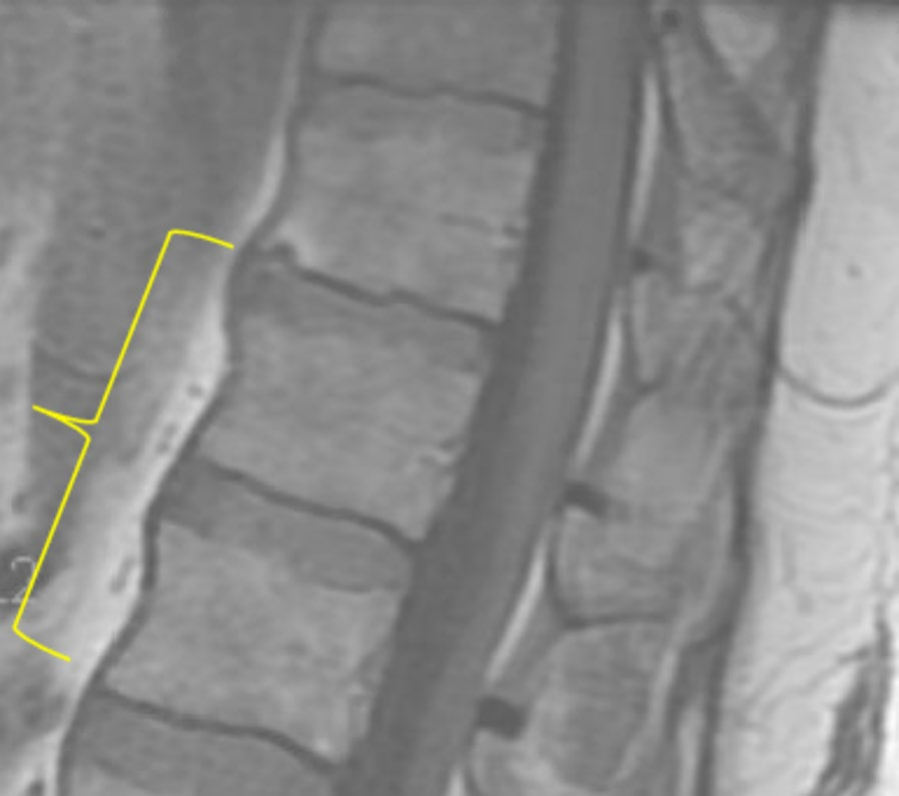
Thoracolumbar spine MRI during the patient’s previous hospitalization, which showed mildly enhancing central T11–T12 intervertebral disc with adjacent endplate enhancement suggestive of early discitis. No discrete epidural collection or abscess identified
